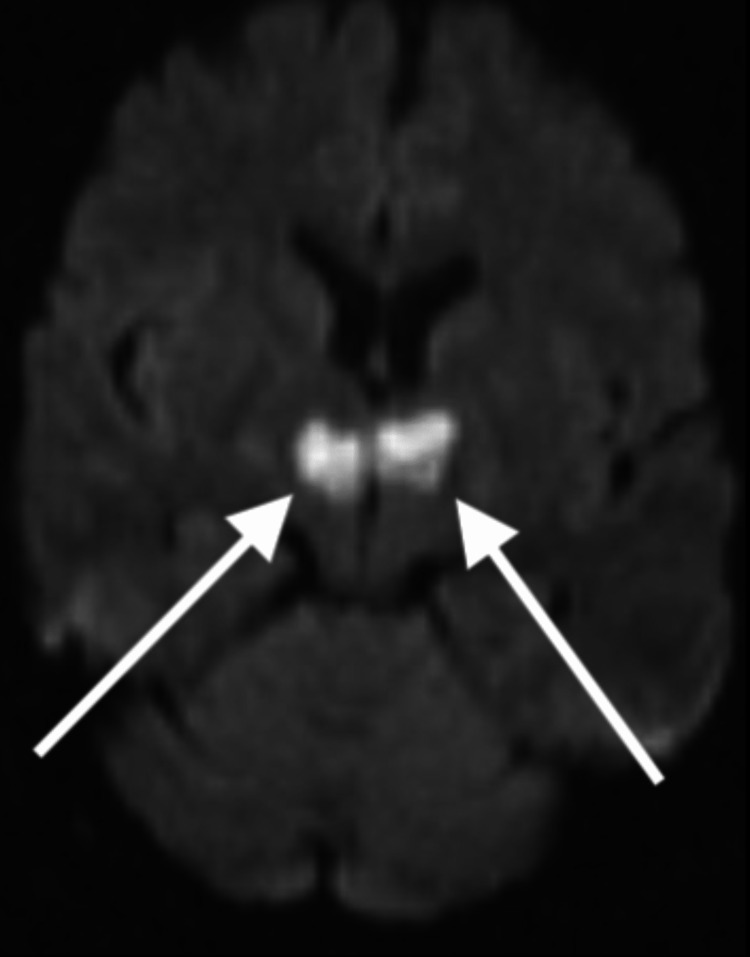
Magnetic resonance imaging of the brain, axial view: diffusion-weighted imaging showing restricted diffusion in both thalami, suggestive of infarcts.
